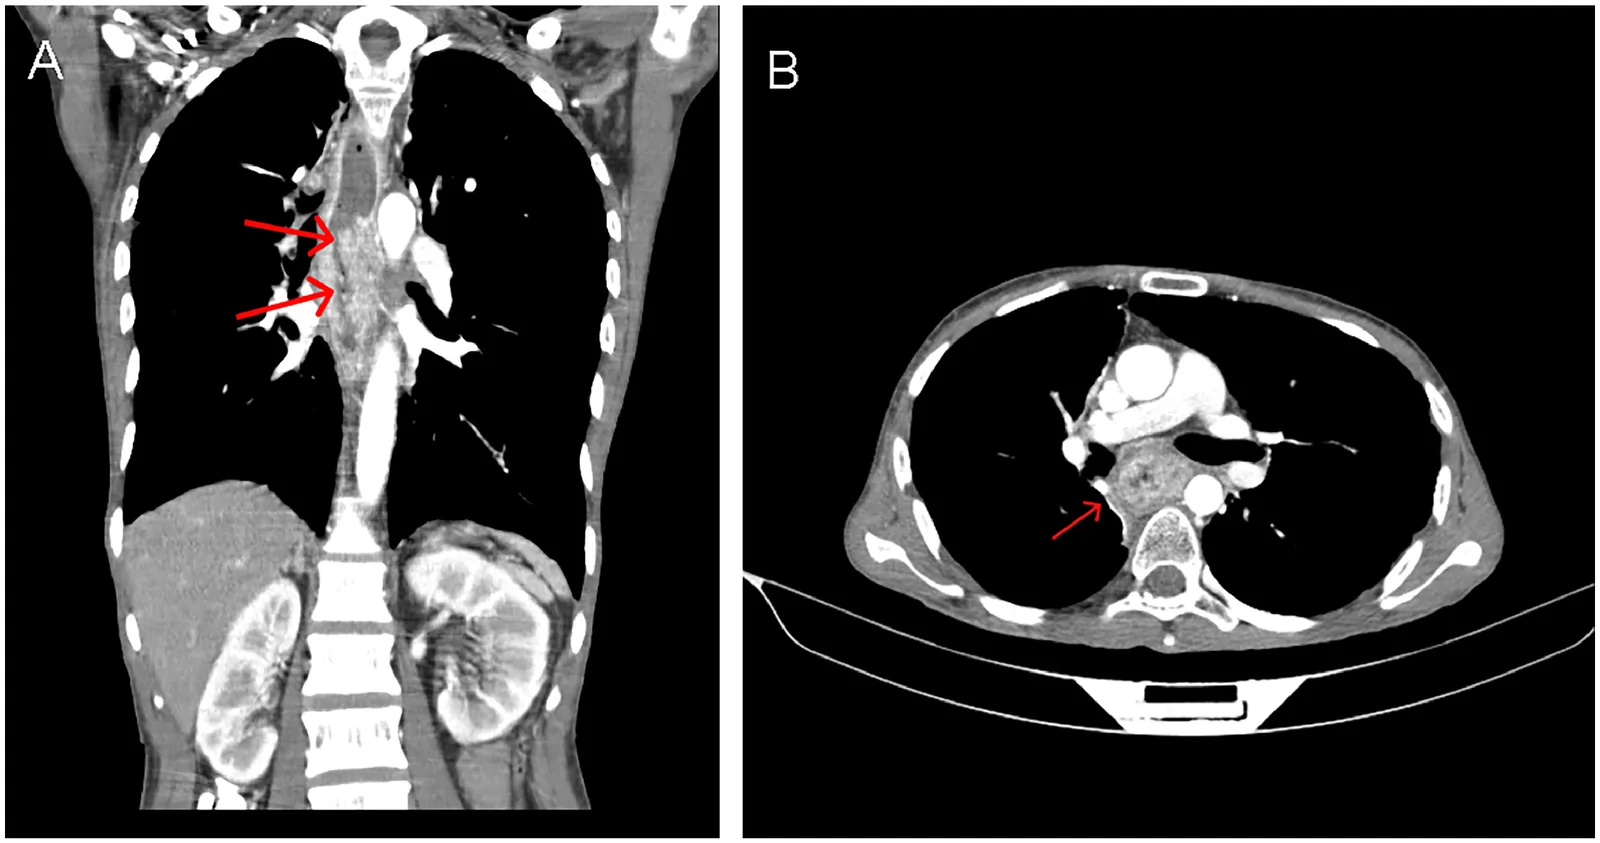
Contrast-enhanced chest computed tomography. Left image: coronal reconstruction demonstrates elongated irregular mid-esophageal soft-tissue thickening/mass in the subcarinal region (red arrows). Right image: axial image through the same level demonstrates the esophageal soft-tissue central airways (red arrow). A definite direct fistulous tract is not shown on the available computed tomography images.
